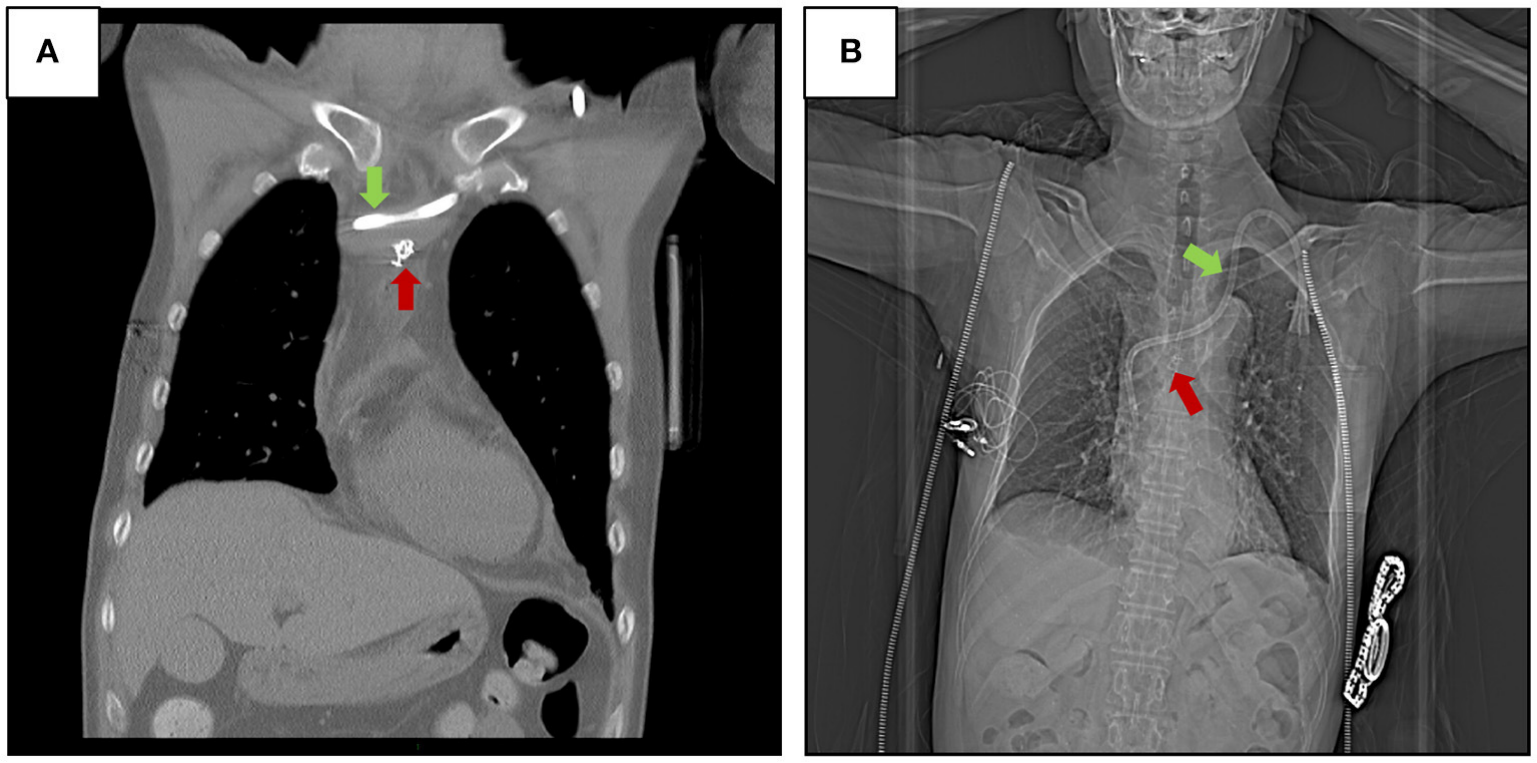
Two embolization microcoils (COOK d ≈ 5 mm) were inserted to block the vascular rupture (A), and X-ray revealed that the cuff catheter and embolization microcoil were appropriately located in the brachiocephalic vein (B).
